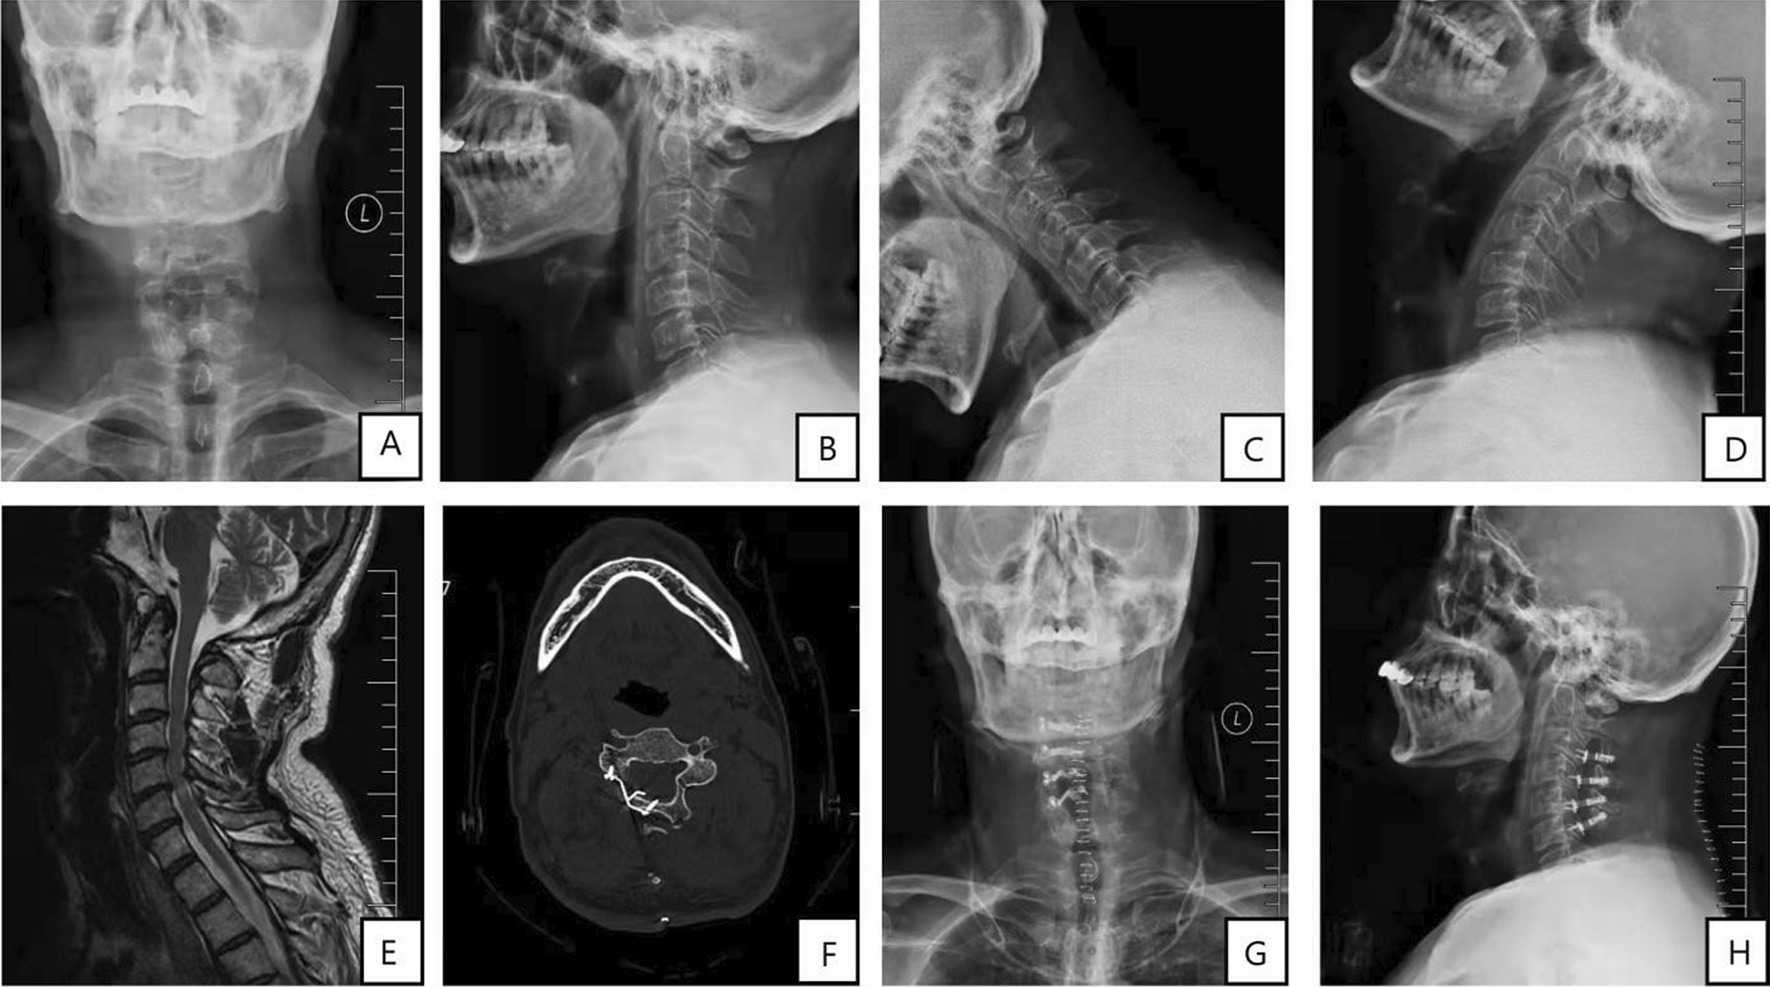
LAMP treatment for multisegments CS. A Preoperative anteroposterior radiograph. B Preoperative lateral radiograph. C–D Preoperative hyperextension and flexion radiograph. E Preoperative cervical MR. F Postoperative CT. G Postoperative anteroposterior radiograph; and H Postoperative lateral radiograph
